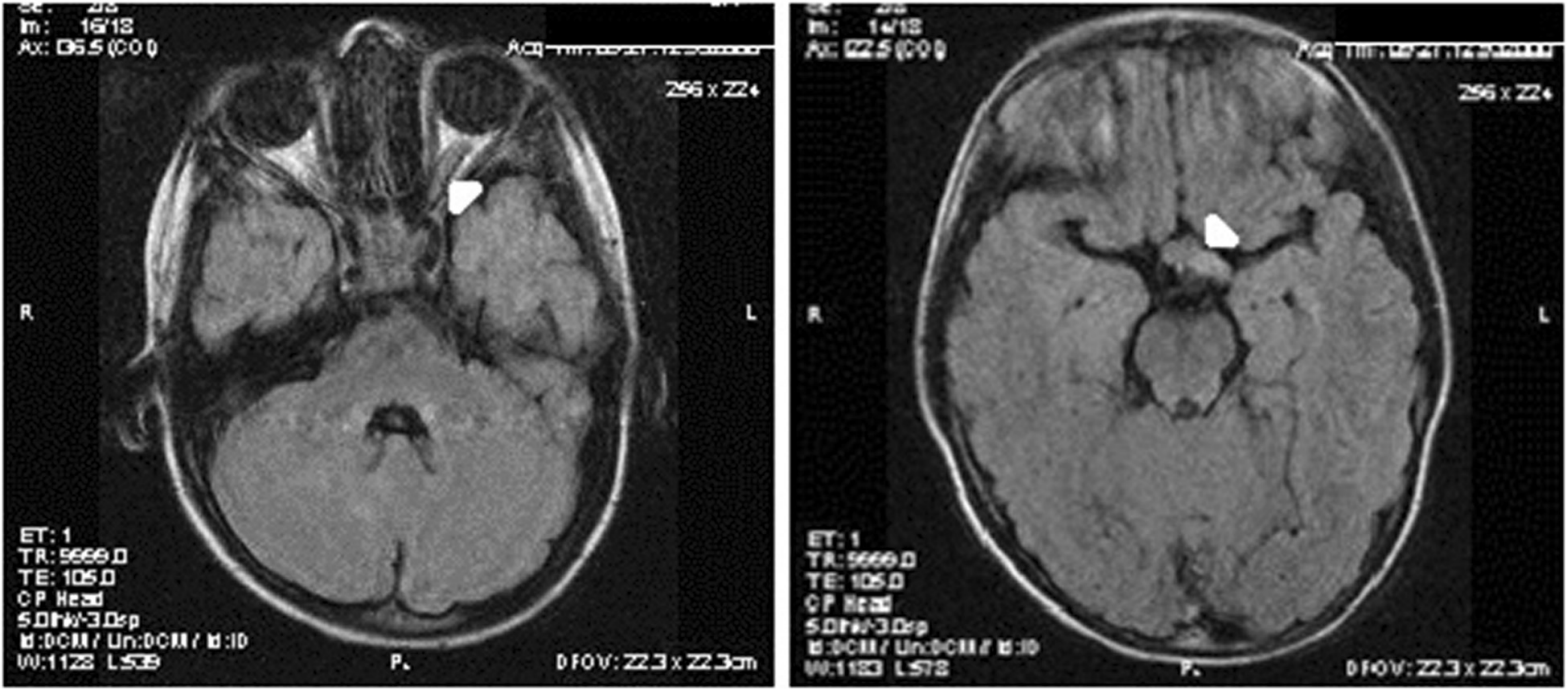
MRI showing optic glioma affecting the optic chiasm; left - thickening of the optic nerve (arrowhead), right - glioma at the optic chiasm (arrowhead)
